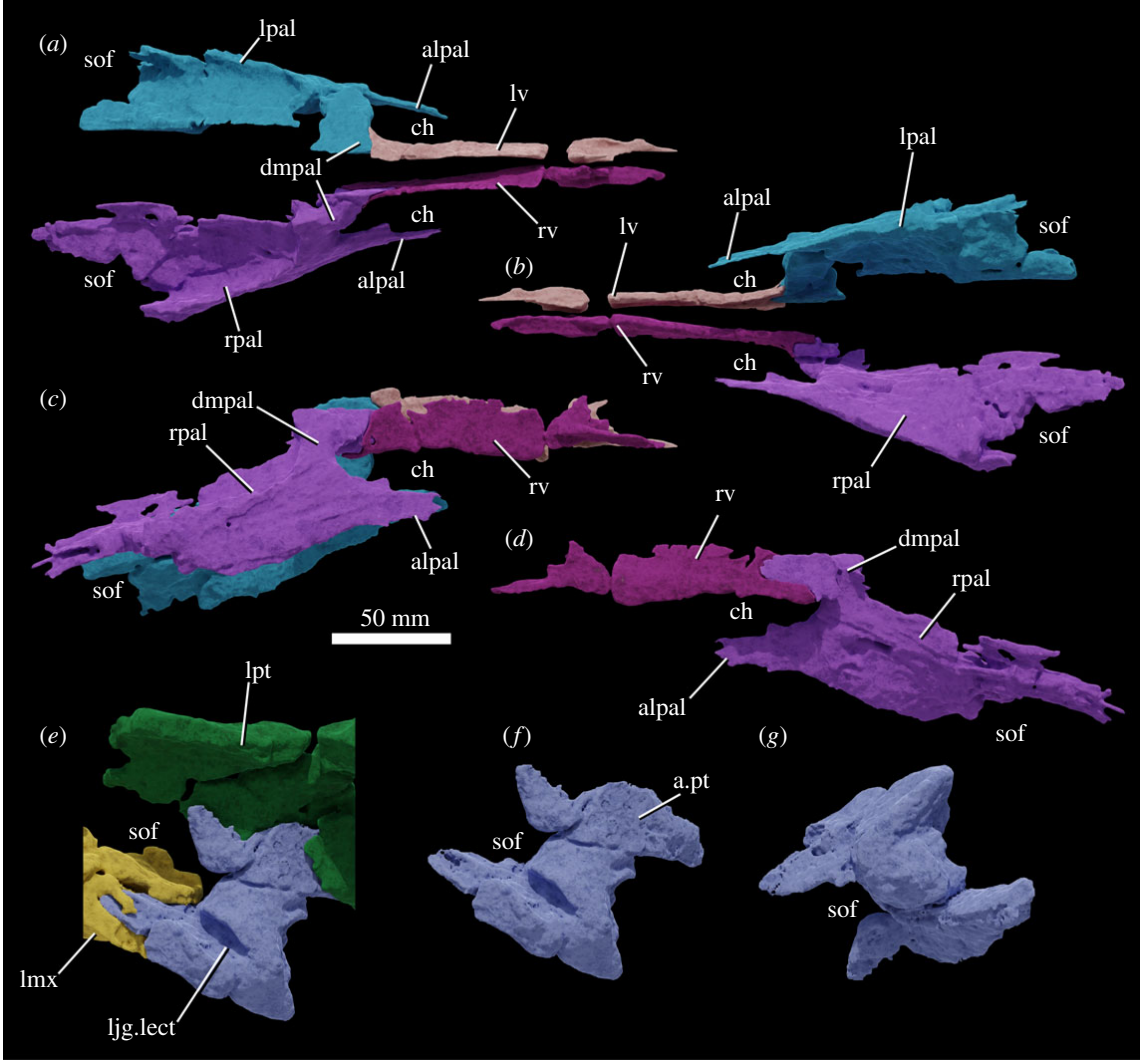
Vomers, palatines and left ectopterygoid-jugal of NHMUK R36620, holotype of *Mambawakale ruhuhu*, reconstructed from CT data. Articulated palatines and vomers in dorsal (*a*), ventral (*b*), and right lateral (*c*) views. Right palatine and vomer in medial (*d*) view. Dorsolateral view of the left ectopterygoid-jugal in articulation with the left pterygoid and maxilla (*e*). Left ectopterygoid-jugal in dorsolateral (*f*) and ventromedial (*g*) views. Abbreviations: alpal, anterolateral process of the anterior end of the palatine; a.pt, articular surface for the ventral process of the pterygoid; ch, choana; dmpal, dorsomedial process of the anterior end of the palatine; ljg-lect, left jugal and left ectopterygoid; lmx, left maxilla; lpal, left palatine; lpt, left pterygoid; lv, left vomer; rpal, right palatine; rv, right vomer; sof, suborbital fenestra.
